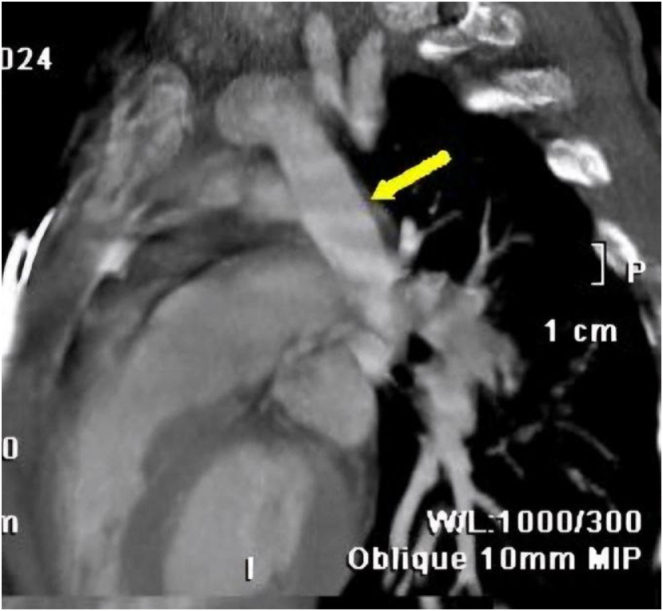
Preoperative CTA image showing the ascending vertical vein (The yellow arrow) draining the left upper pulmonary vein.
